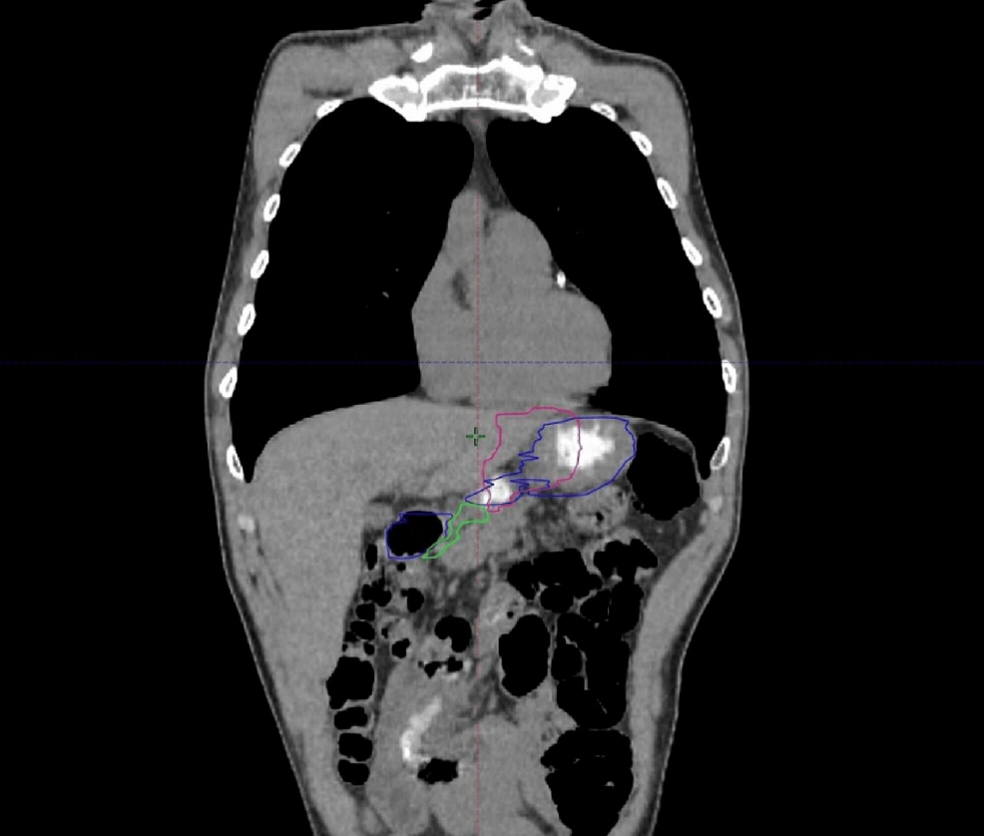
CT image of the patient (coronal view) A coronal representative image of the RGEA (green structure) running along the greater curvature of the stomach (blue) in its right aspect. Note the proximity between the RGEA and the PTV (pink) as in this patient the celiac nodes were covered in the target volume.
